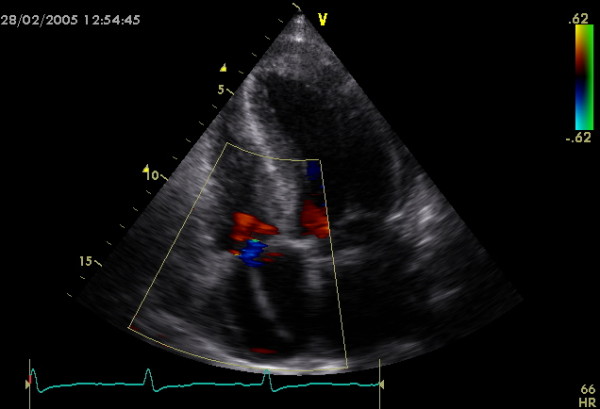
Implantable Cardioverter Defibrillator wire in a Hypertrophic Cardiomyopathy patient. An echocardiographic apical four chamber view showing the wire of an Implantable Cardioverter Defibrillator in the right ventricle, passing through tricuspid valve. Defibrillator was implanted in a male HCM patient presenting with previous syncope episodes and positive family history for sudden death.
